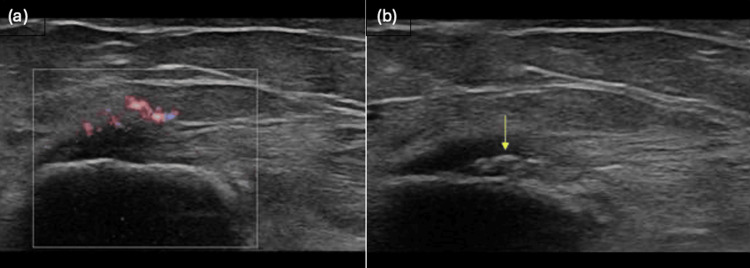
Ultrasound images showing reactive hyperaemia (panel 2a, left) and intratendinous calcification (panel 2b, right) in a patient with medial epicondylitis Credit: [17]
